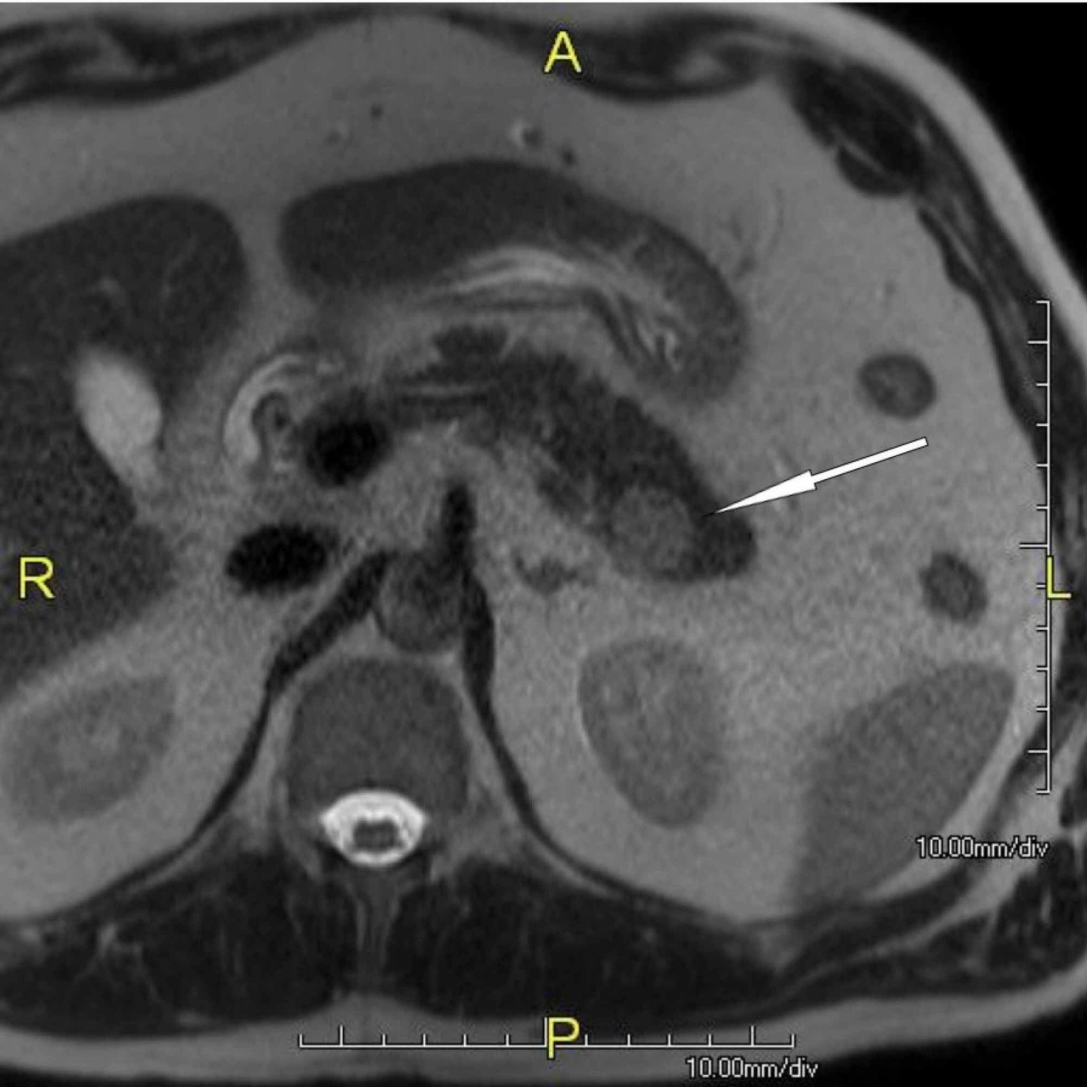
MRI of the 2-cm tumor in the pancreatic tail (intrapancreatic spleen) Intrapancreatic accessory spleen (arrow) on axial, fat-saturated, T2-weighted MR image, which shows high signal intensity compared to pancreas MRI: magnetic resonance imaging
